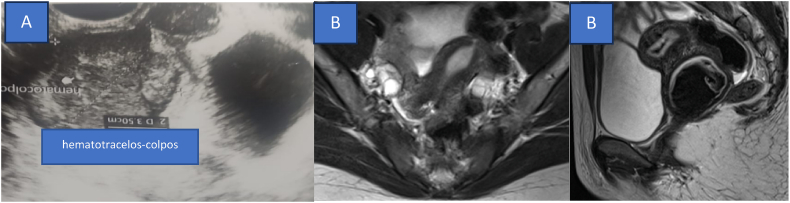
(A). Abdominal ultrasonography showing echo intensity in cervix suggested hematocolpos. (B) MRI showing hyperintense cystic lesions in T1W1 and hypointense in T2W1 and vaginal distal was not visible agenesis distal vaginal with hematocolpos.
